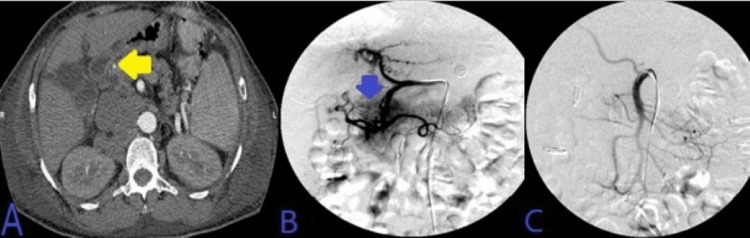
A: CT scan with contrast of the abdomen showed hypertrophic submucosal arterioles along the second portion of the duodenum compatible with oozing seen on endoscopy. B: Selective celiac angiograms demonstrated irregularly tortuous duodenal arterioles as the culprit of bleeding from branch of the GDA with shunting toward the SMA, therefore no coils were used at this time to avoid non-target migration and bowel ischemia. C: Super-selective GDA angiograms with embolization using particulate polyvinyl alcohol (PVA) 500 was done. Post-embolization selective celiac and SMA angiograms demonstrated patent jejunal and ileocecal and colic arteries with replaced right hepatic artery with no signs of backflow duodenal blushes or bleeding. CT: computed tomography; GDA: gastroduodenal artery; SMA: superior mesenteric artery.
